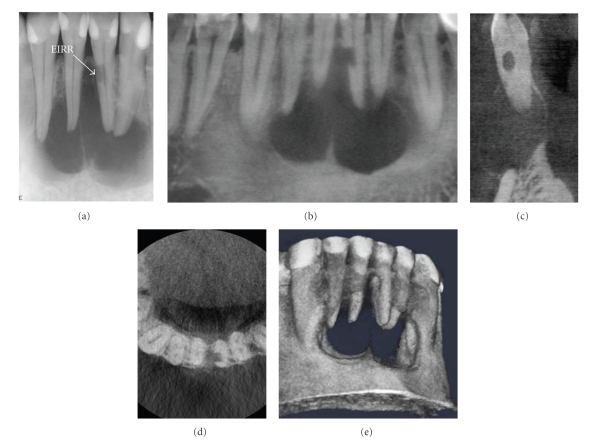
After suffering a traumatic blow from a soccer ball six years earlier, a 28-year-old male presented with a soft, convex-shaped indurated buccal and lingual swelling in the mandibular symphyseal region. A periapical image (a) showed a large, multilocular lesion. External inflammatory root resorption (EIRR) was noted on the mandibular left lateral incisor. All four mandibular anterior teeth tested nonvital. CBCT images ((b) curved planar, (c) cross-sectional, (d) axial, (e) 3D reconstruction) showed that the resorptive lesion was extended from the root canal space to the periodontal membrane, necessitating repair or extraction; no exploratory procedure was necessary to determine the extent of the defect.
